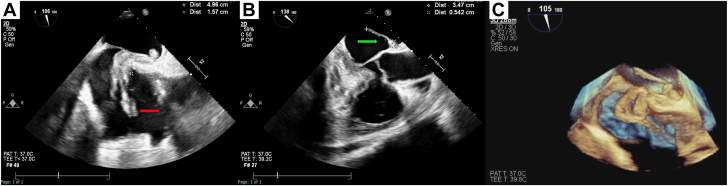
Transesophageal echocardiogram (TEE) images. (A) TEE showing thrombus in the right atrium (red arrow) crossing the PFO and extending into the left atrium. (B) TEE showing thrombus in the left atrium (green arrow). (C) Three-dimensional TEE showing clot in transit lodged in the patent foramen ovale.
